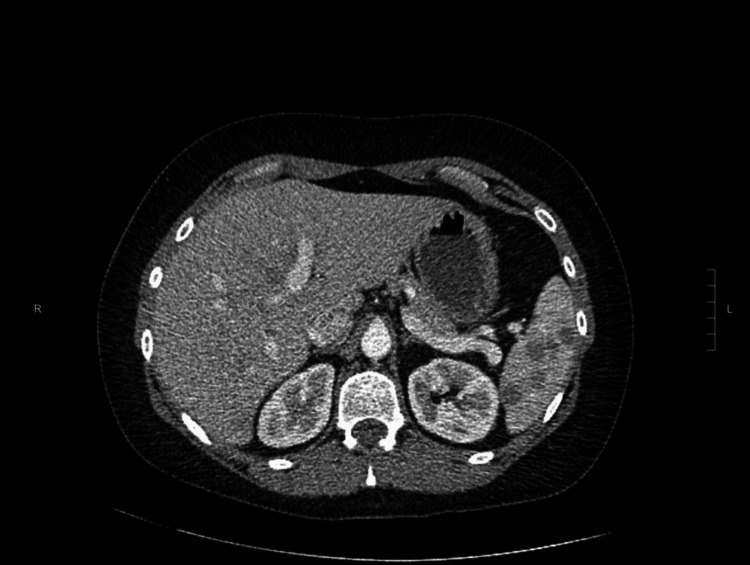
Computed tomography of the abdomen and pelvis with IV contrast showing low-attenuation lesions in the liver and spleen
